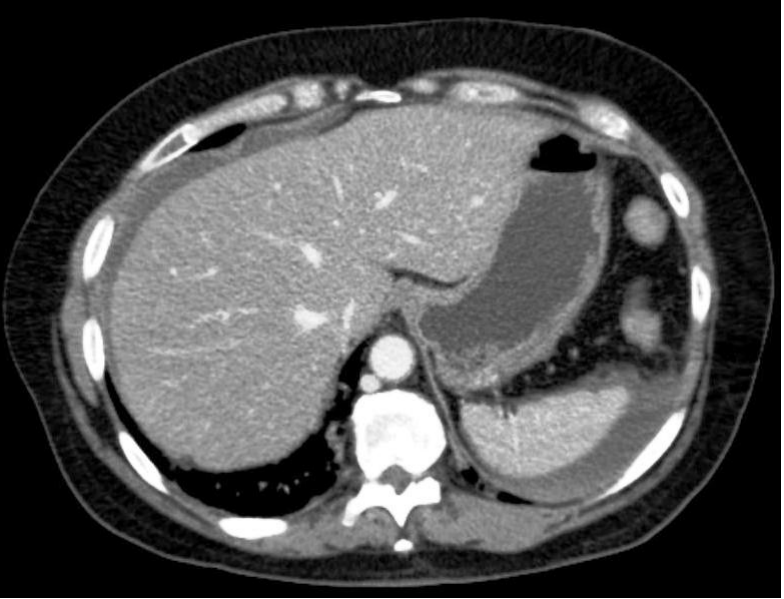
Abdominal computed tomography (CT) scan (axial) with intravenous contrast demonstrating an important hemoperitoneum with densities around the spleen and the right lobe of the liver.
